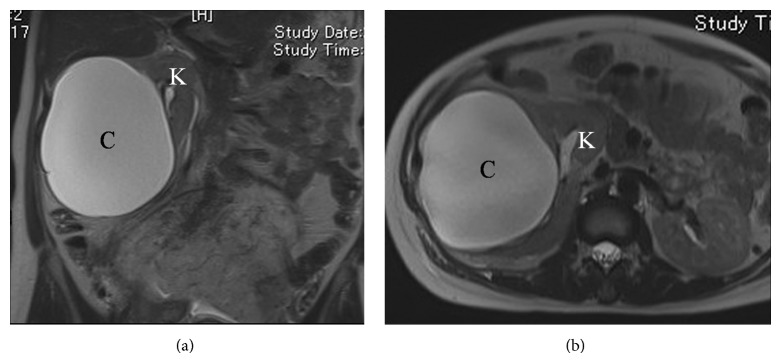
Magnetic resonance imaging (T2-weighted image) of a large cystic mass arising from the lower pole of the right kidney. (a) Coronal and (b) axial view. C, cystic mass; K, right kidney.
